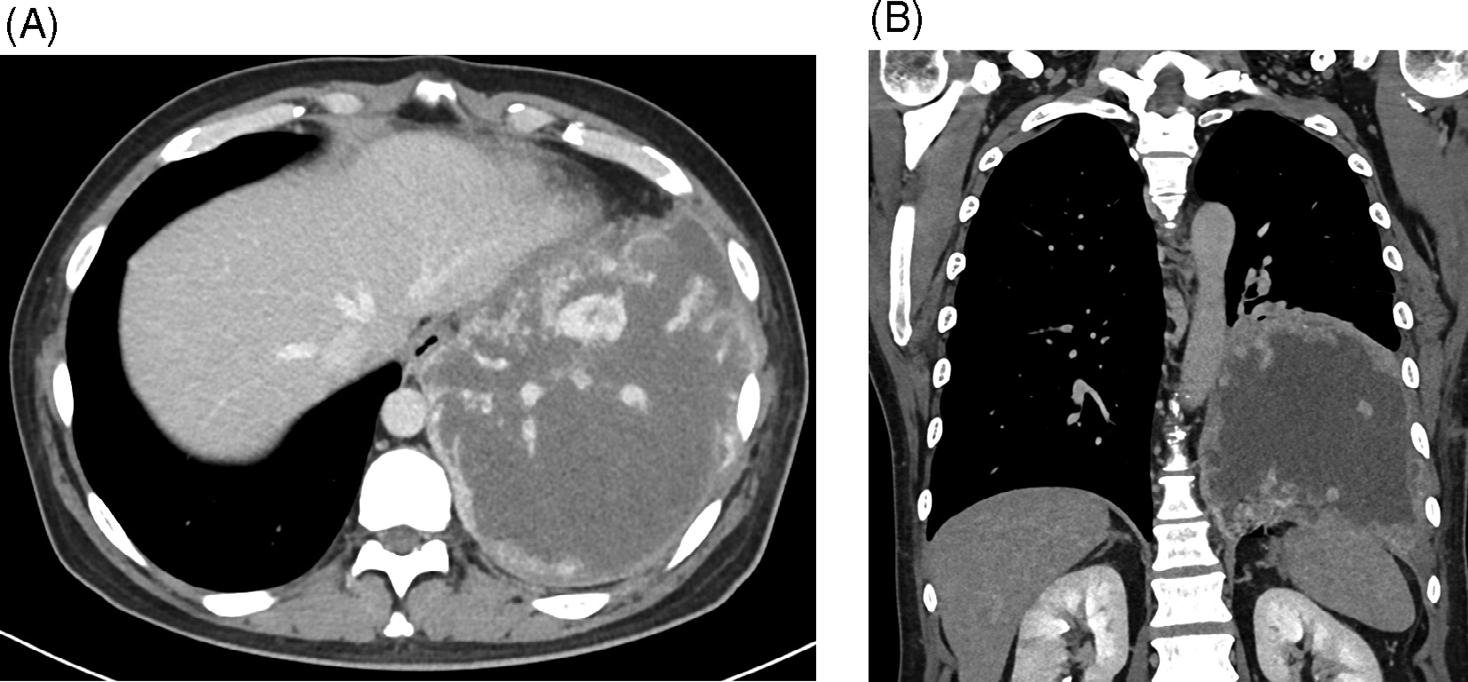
(A) Chest axial image computed tomography (CT) demonstrated 15 × 14 cm sized mass in the left lower lobe in the lung. (B) Coronal CT images. The circumference of the mass showed a high contrast enhancement similar to that of blood vessel.
